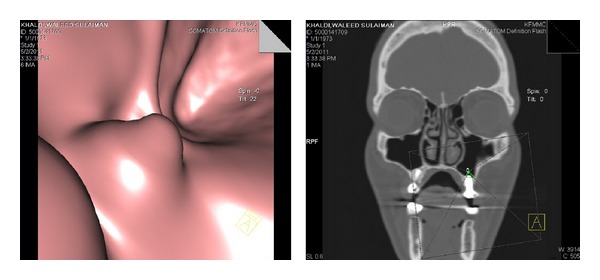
The CT and DentaScan reformatted flythrough image of the staged osteotome sinus floor elevation showing the intact Schneiderian membrane over the projection of the apical border of the first implant.
